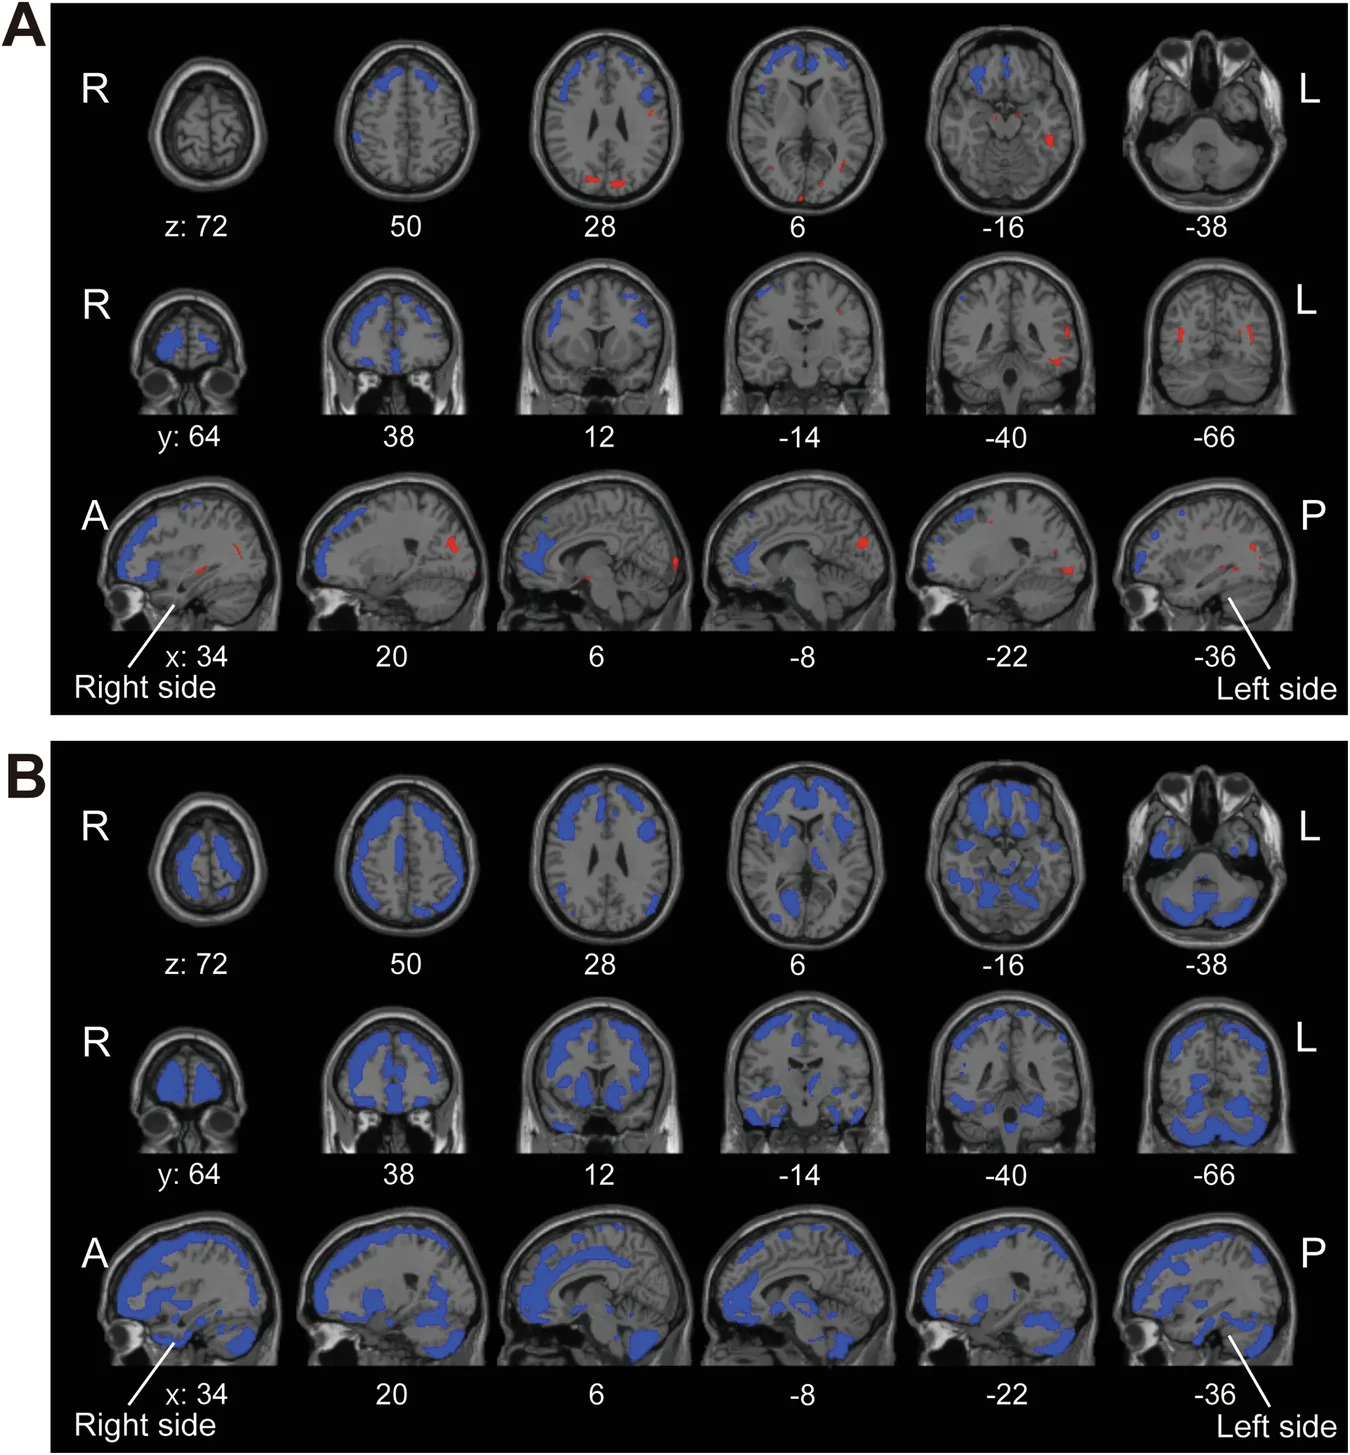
Commonly affected areas across psychiatric disorders. A Common brain regions showing reduced (blue) and increased (red) SUVR_30–50 min_WB, respectively, in patients with schizophrenia, bipolar disorder and ASD compared with healthy participants. B　Common brain regions showing reduced (blue) SUVR_30–50 min_WM, in patients with schizophrenia, bipolar disorder and ASD compared with healthy participants. MNI coordinates for the z-axis (axial slices), y-axis (coronal slices) and x-axis (sagittal slices) were shown bottom of each slice.
